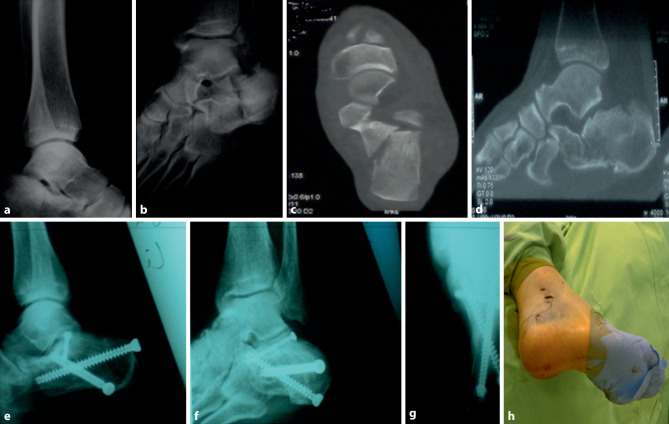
Patient case. Patient with intra-articular calcaneal fracture after fall from a great height. Sanders IIA type fracture, treated with 2‑point distractor fixation technique. a Preoperative lateral X‑ray and b preoperative axial X‑ray. Computed tomography (CT) image c, d). e Postoperative lateral X‑ray shows good reconstruction of Böhler’s angle and Gissane’s angle. f Postoperative oblique X‑ray shows good reduction of calcaneal height. g Postoperative axial X‑ray shows good reduction of calcaneal length. h Intraoperative incision picture
